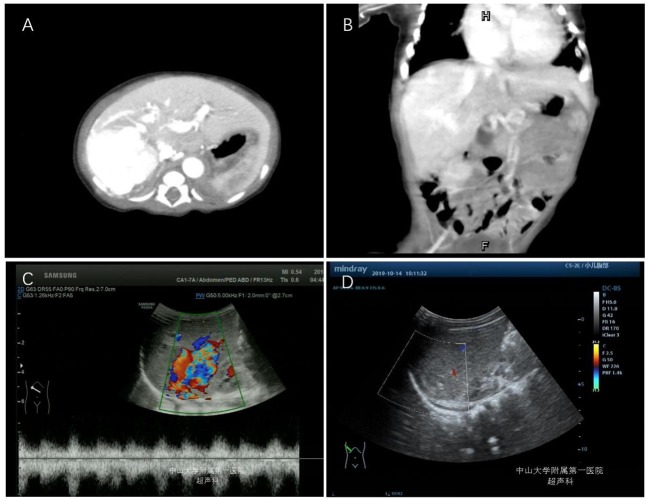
Enhanced CT scan and color Doppler examinations of the liver in case 2. (A,B) CT images of the infant at 3 days old. (C) Color Doppler analysis of the liver at 3 months after metoprolol treatment. (D) Color Doppler analysis of liver at 8 months after metoprolol treatment.
